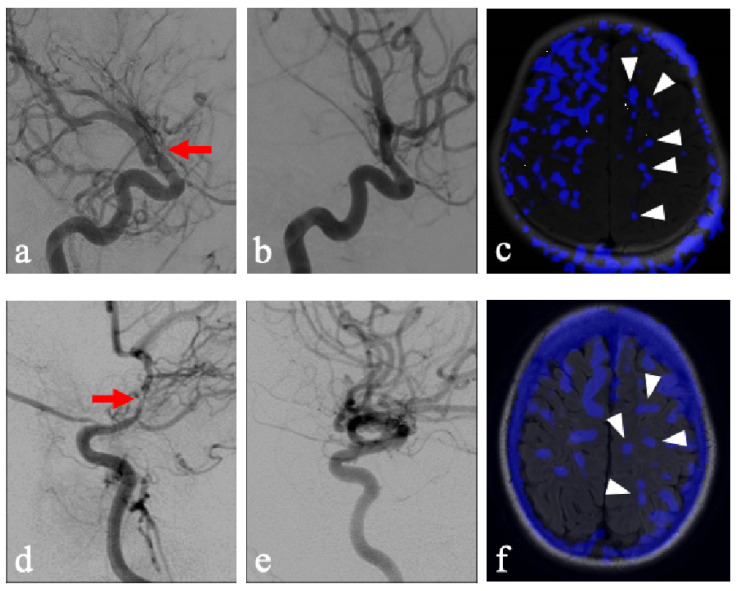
Two pediatric patients with unilateral vaso-occlusive disease of the right anterior circulation. (a) A right-sided ICA DSA with severe stenosis of the supraclinoid ICA (red arrow) corresponding to the FLH. (b) A left-side ICA DSA corresponding to the NFLH hemisphere. (c) Axial T2/FLAIR MRI with areas of steal phenomenon overlaid in blue, demonstrating the occurrence of steal within the NFLH (white arrowheads). The Fazekas for the right and left hemisphere were graded independently as 0 and 0. The steal for the right and left hemisphere were graded as 2 and 1, respectively. (d) A right-sided ICA DSA with severe stenosis of the supraclinoid ICA (red arrow) corresponding to the FLH. (e) A left-side ICA DSA corresponding to the NFLH hemisphere. (f) Axial T2 MRI with areas of steal phenomenon overlaid in blue, demonstrating the occurrence of steal within the NFLH (white arrowheads). The Fazekas for the right and left hemisphere were graded independently as 3 and 2, respectively. The steal for the right and left hemisphere were graded as 3 and 3, respectively.
